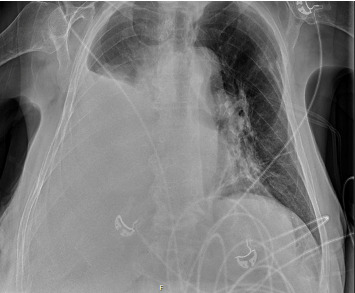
Chest X-ray showed a right-sided pleural effusion tracking to the apex with associated complete lower and middle lobe atelectasis, as well as partial atelectasis of the right upper lobe.
